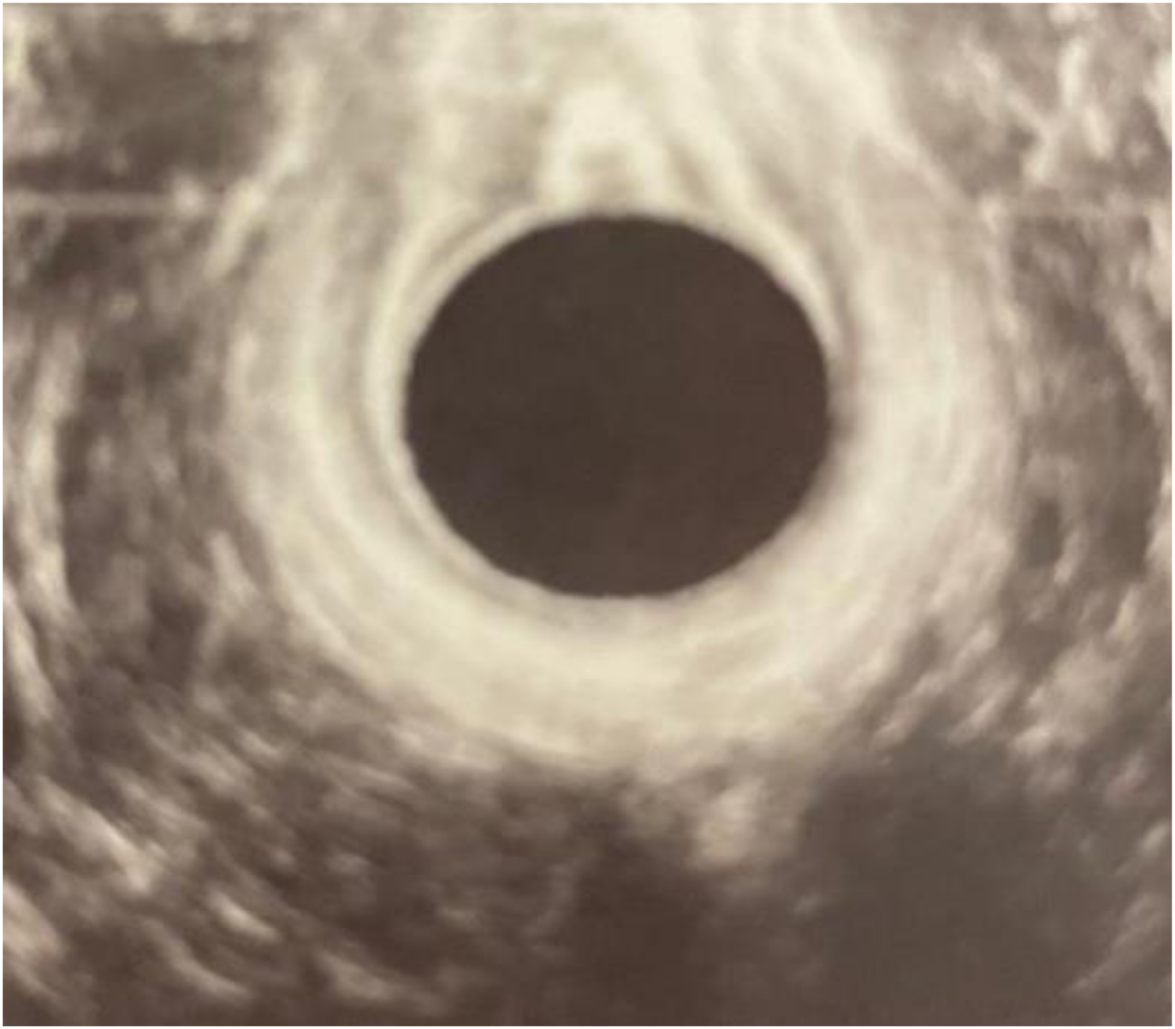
Endoanal ultrasound. The opening of the fistula on the anterior wall was shown on the ultrasound picture above the probe (black circle).
